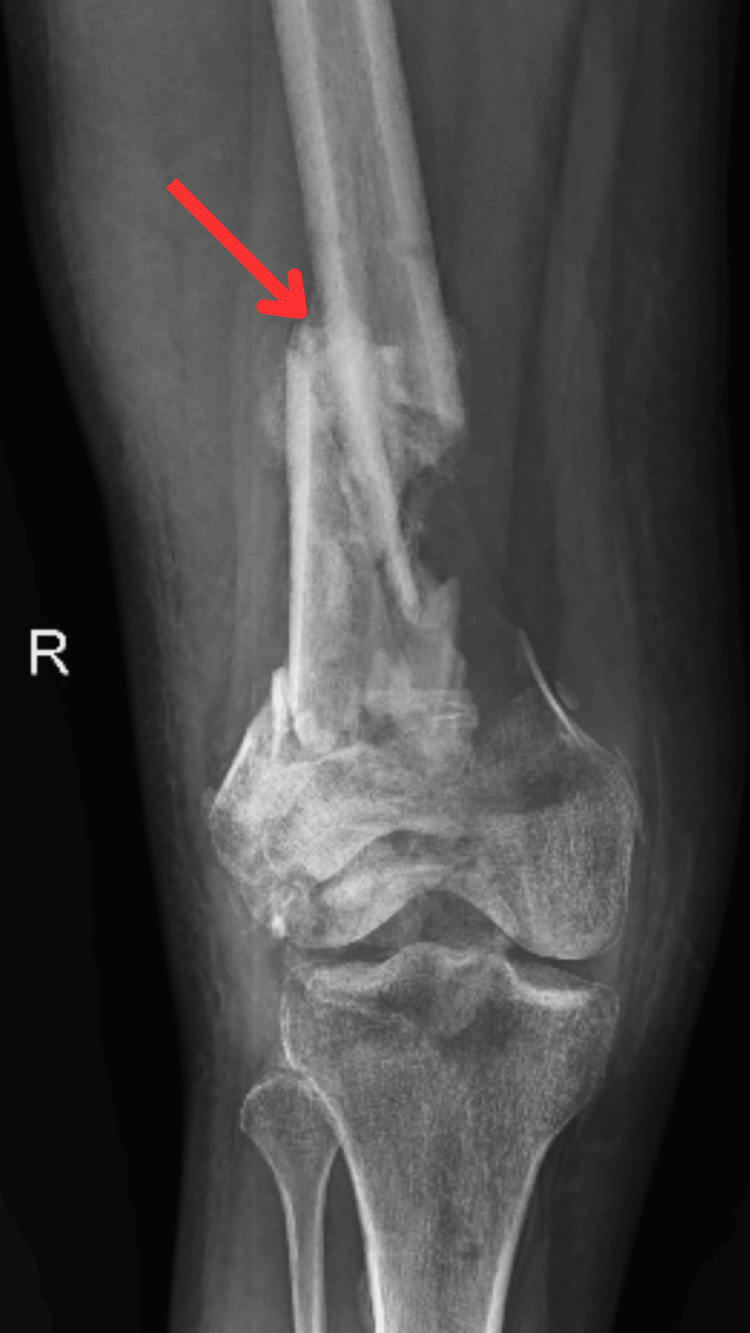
X-ray of comminuted distal femur fracture (anteroposterior view) The red arrow points to the fracture site.
